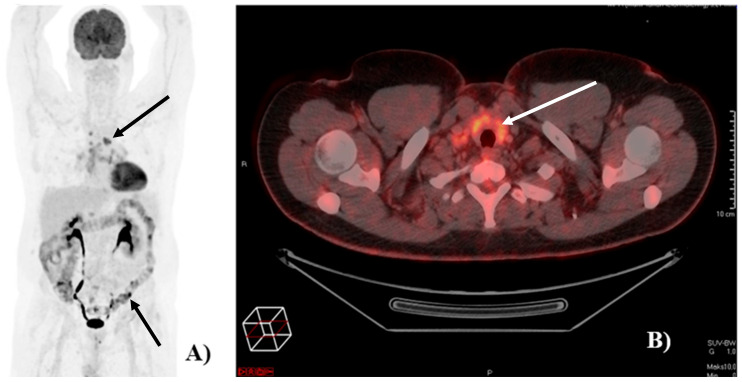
Image of [18F]FDG-PET/CT-detected immune-related adverse events (irAEs). (A) Example of a patient with [18F]FDG-PET/CT-detected irAEs in the colon and in mediastinal lymph nodes (black arrows). (B) Example of a patient with [18F]FDG-PET/CT detected irAE in the thyroid (white arrow).
